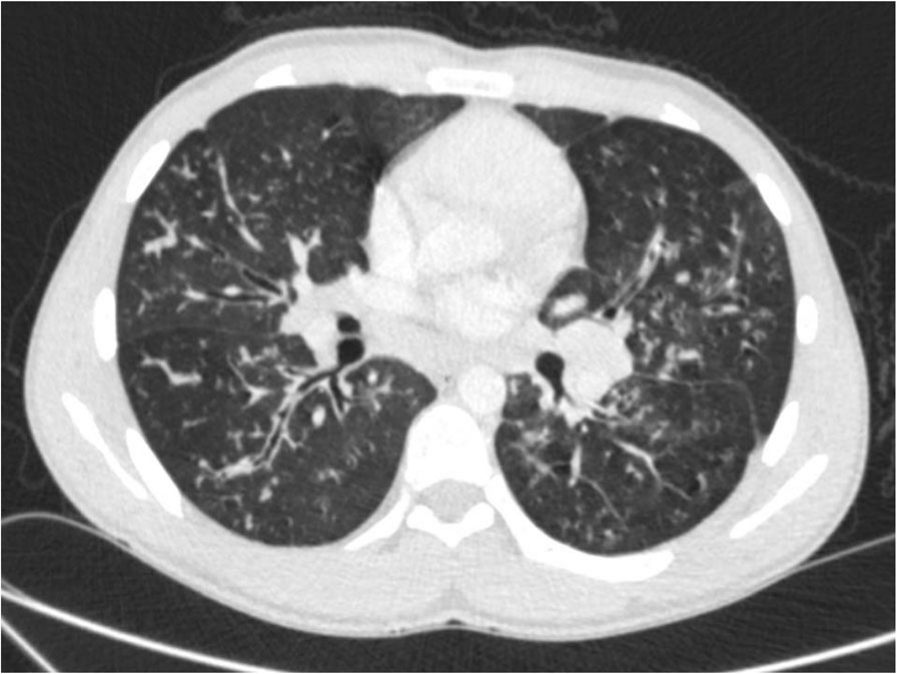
Computed tomography of chest showing bronchiectasis changes and peribronchovascular thickening seen throughout both lung fields. Tree in bud appears in the periphery predominantly in the right middle and lower lobe and lingular segment of the left upper lobe
